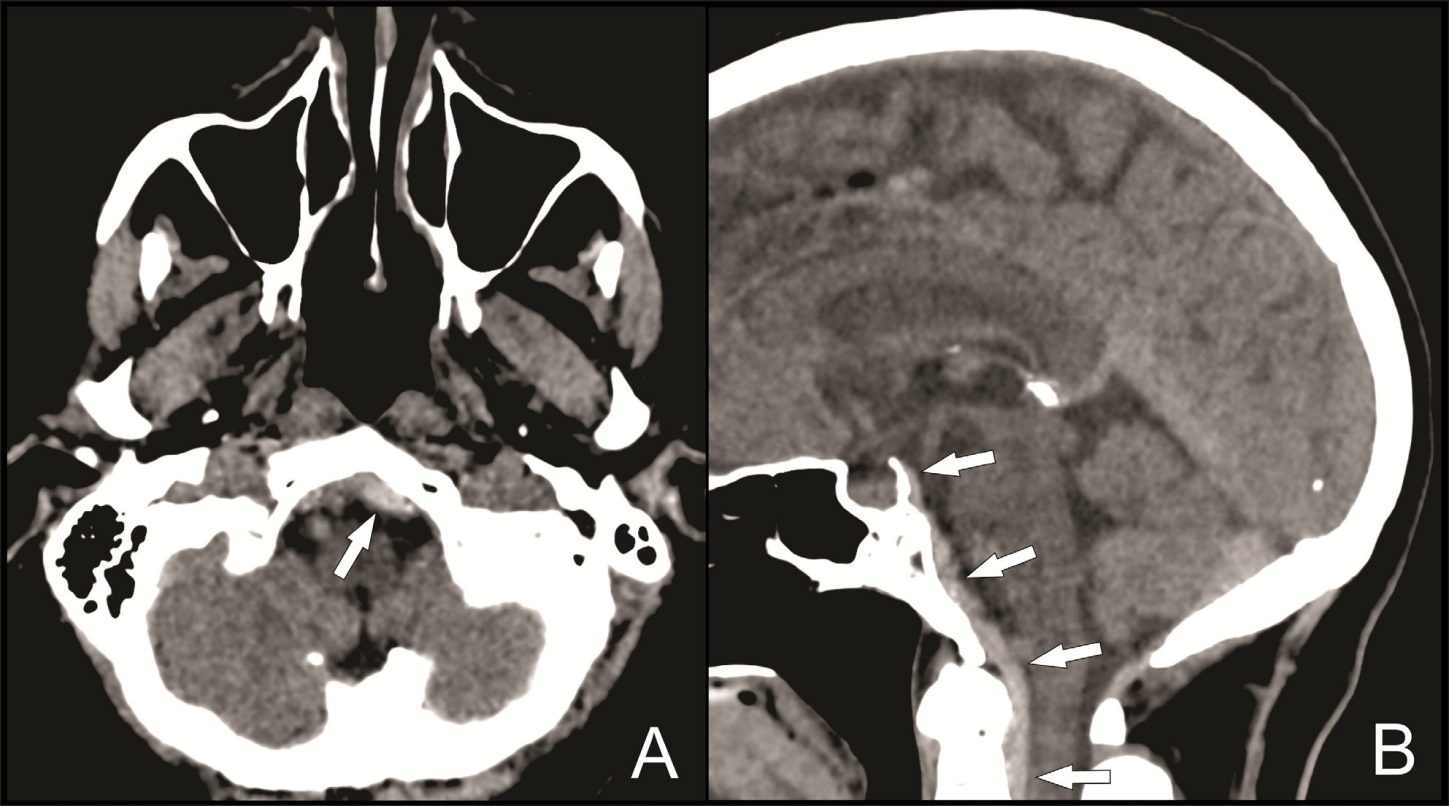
Magnetic resonance imaging (MRI) of the cervical spine was then performed to assess this collection better. MRI showed a hematoma posterior to the tectorial membrane descending anteriorly into the spinal canal up to the posterior body of C6, best seen on T1-weighted images (Figure 3A, white arrows), and an intact tectorial membrane, best seen on T2-weighted images (Figure 3B, white arrowhead). These imaging findings are suggestive of a retroclival subdural hematoma.
